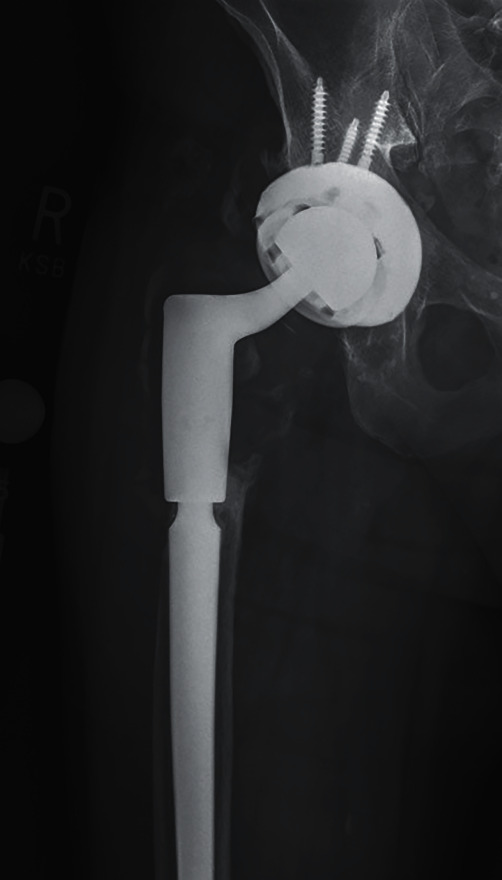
Preoperative anteroposterior X-ray of the right hip.
